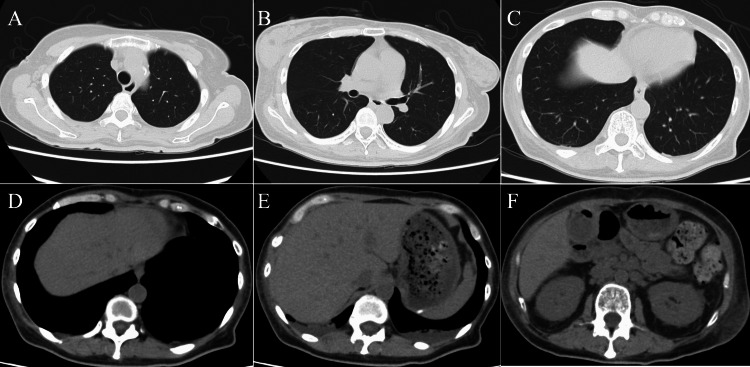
Computed tomography (CT) findings CT showed neither lung (A, upper part; B, middle part; C, lower part) nor liver (D, upper part; E, middle part; F, lower part) metastasis.
